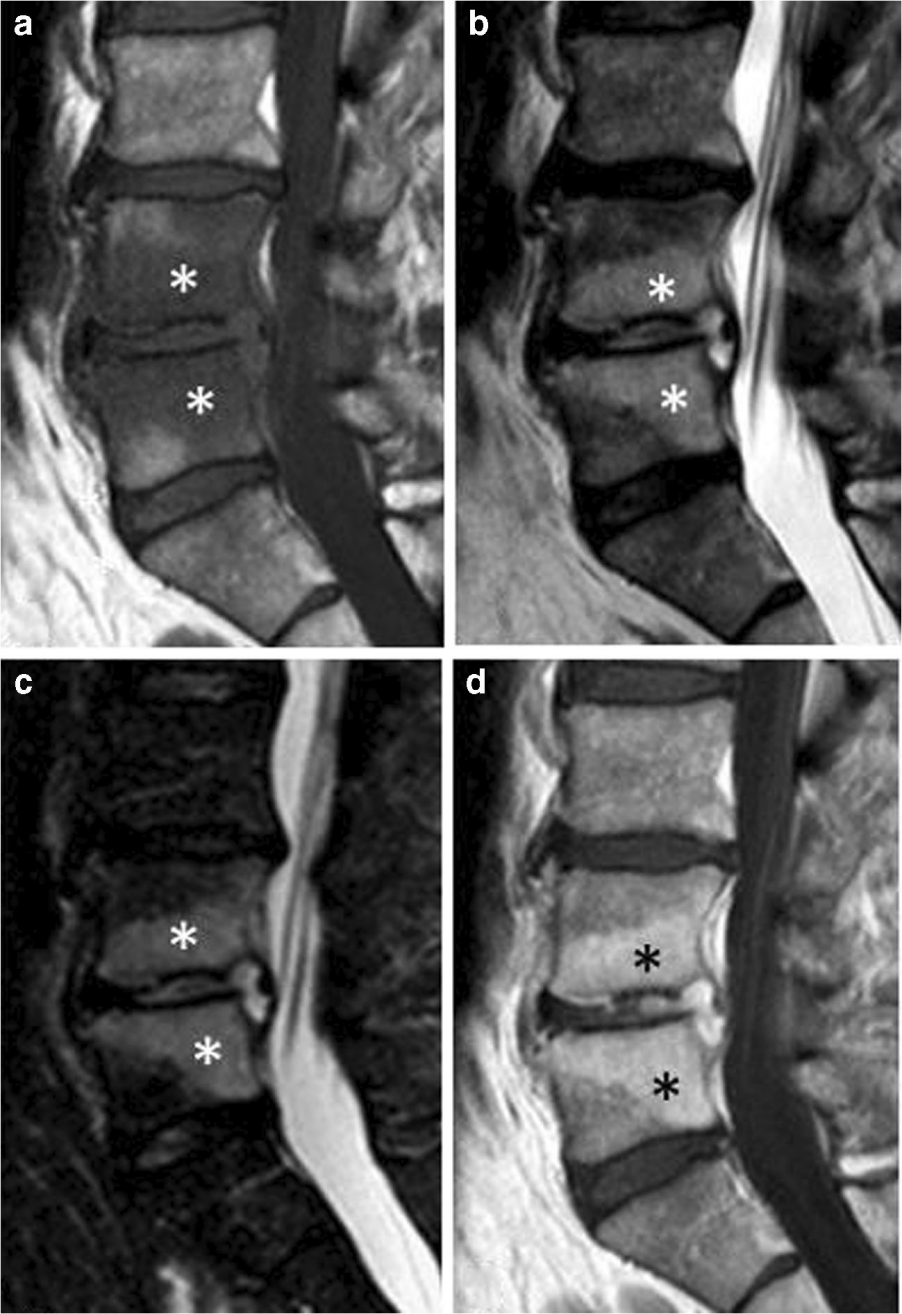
a, b, c, d Infectious. Spondylodiscitis involving L4–L5 in a 52-year-old man with fever and low back pain. MRI shows a large ELMSI (*) of the vertebral bodies, appearing hypointense in T1w imaging (a), hyperintense in T2w (b), STIR (c), and contrast-enhanced T1w imaging (d). The interposed disc is also involved in the inflammatory process, appearing hyperintense on T2w, STIR and contrast-enhanced T1w sequences
